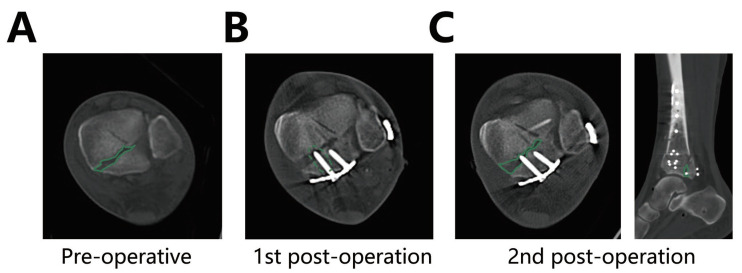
Inappropriate length of the screws impedes Volkmann fragment repositioning. (A) The CT scan shows the morphology of the preoperative pilon fracture bone fragments. (B) The posterior screws should be shorter than the size of the posterior ankle fragment. (C) CT scan of the ankle after the second surgery showed that an excessively long posterior screw impeded the reduction of the anterolateral bone fragments during the second surgery.
